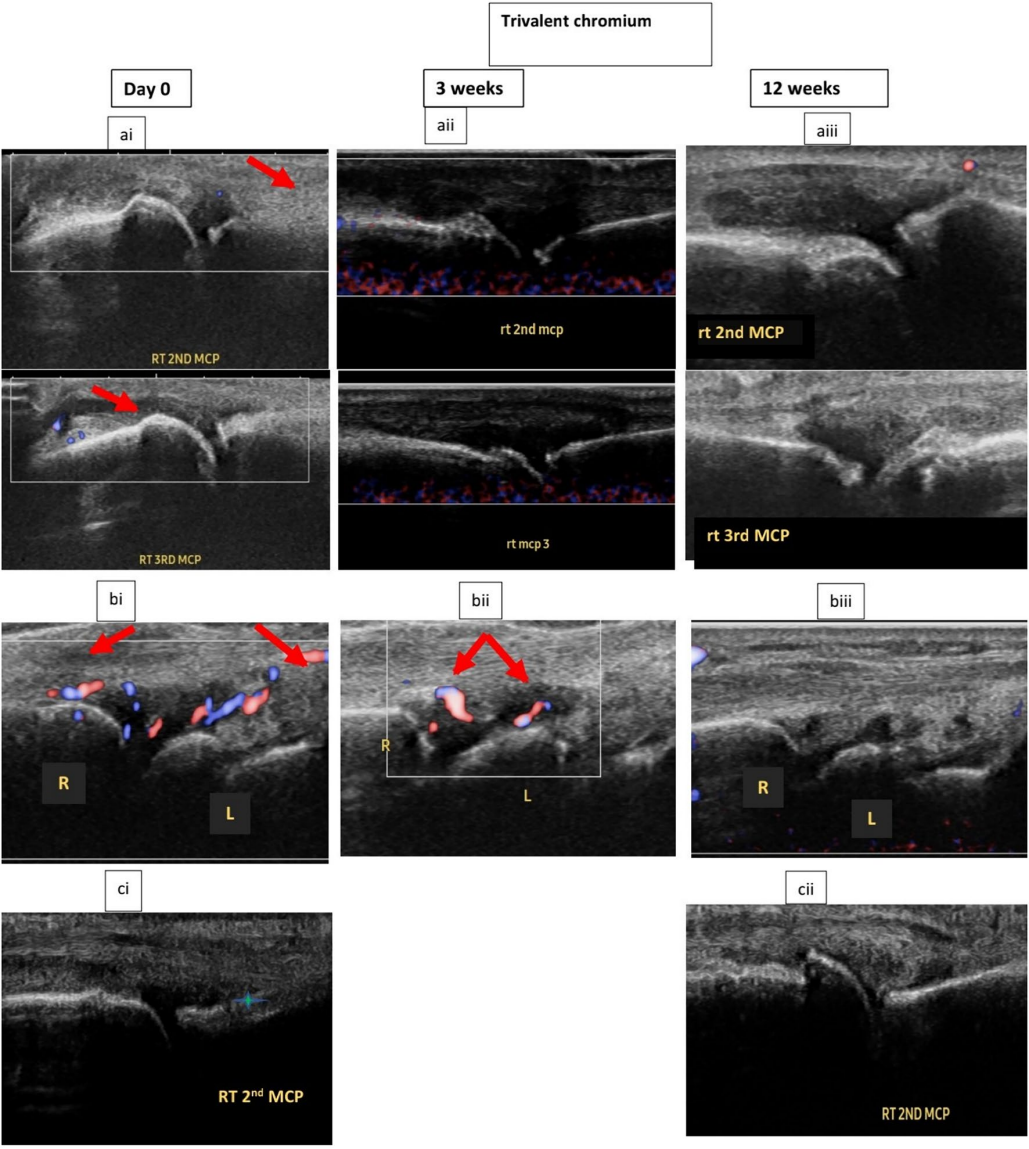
Hands of ultrasound of some patients who received Cr III, ai red arrow indicates PDUS and blue sign indicates effusion.ai Hands ultrasound of the first patient on the first visit revealed that: GLOESS = 49, SH = 49, PDUS = 3 in the right second, third and fourth metacarpophalangeal (MCP) joints, (red arrows showed in the image indicates PDUS in the second and third MCP joints), number of joints effusions = 16. aii Second visit revealed: doppler activity disappeared (PDUS = 0). aiii Third visit’s ultrasound showed: GLOESS decreased = 35, SH decreased = 35, PDUS = 0, effusions disappeared (number of effusions = 0). Bi Hands ultrasound of the second patient revealed that: bi First visit GLOESS: 52, SH = 52, PDUS = 5 (doppler activity was present in left radio-lunate (RL) and intercarpal joints) (red arrow shows PDUS in the left RL joint), number of effusions = 11. bii On the second visit, PDUS decreased. biii On the third visit, GLOESS decreased = 41, SH decreased = 41, PDUS decreased = 2, number of effusions decreased = 4. ci Ultrasound of the first visit showed that: GLOESS = 55, SH = 55, number of joints effusions = 16. Cii: Ultrasound of the third visit showed that: GLOESS decreased = 31, SH decreased = 31, number of effusions decreased = 2. (The blue sign shows that effusion in the second MCP ci that was present on the first visit disappeared in cii on the third visit
